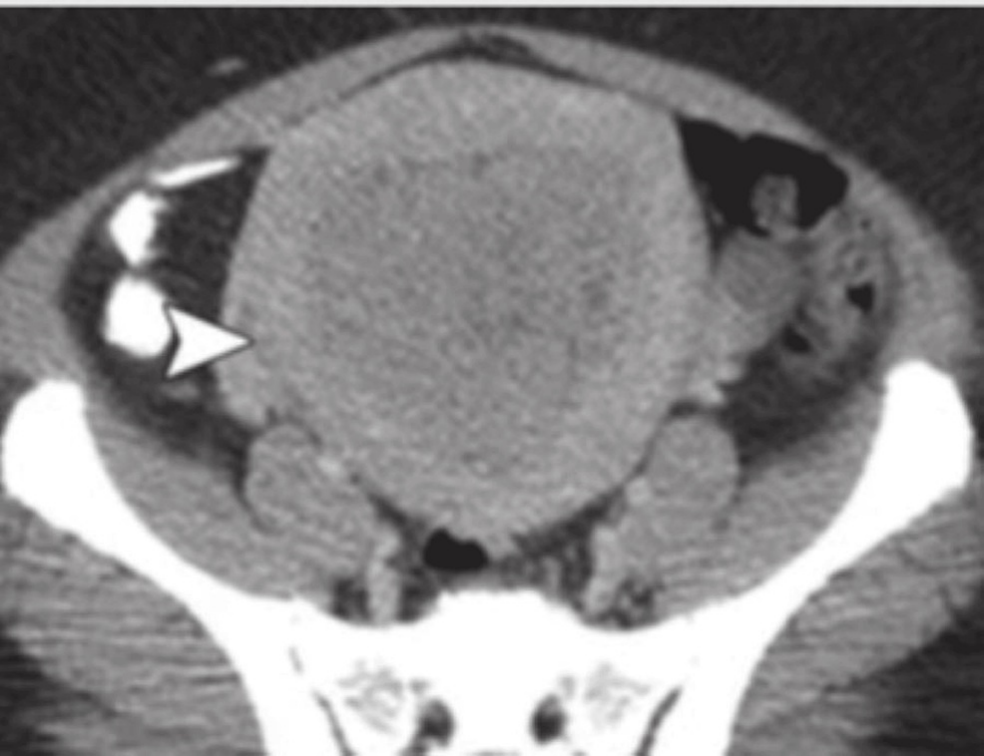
Axial CT of the patient The arrow shows the bulky uterus with no parametrial invasion. CT: Computerized tomography
